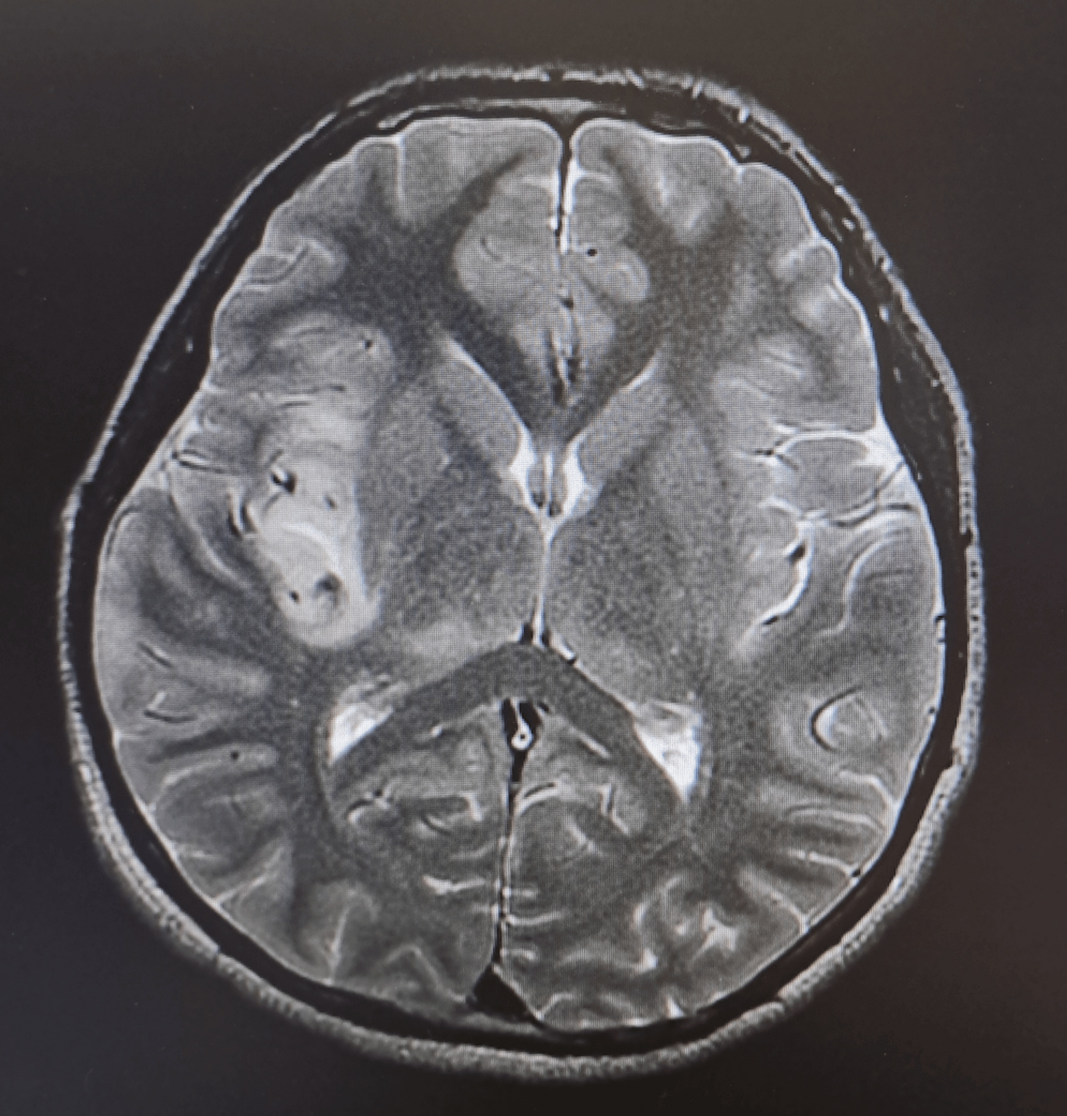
Axial T2-weighted MRI of the brain upon admission. It reveals hyperintensity cortical swelling over the medial temporal insula with few cerebral foci of hemorrhage.
